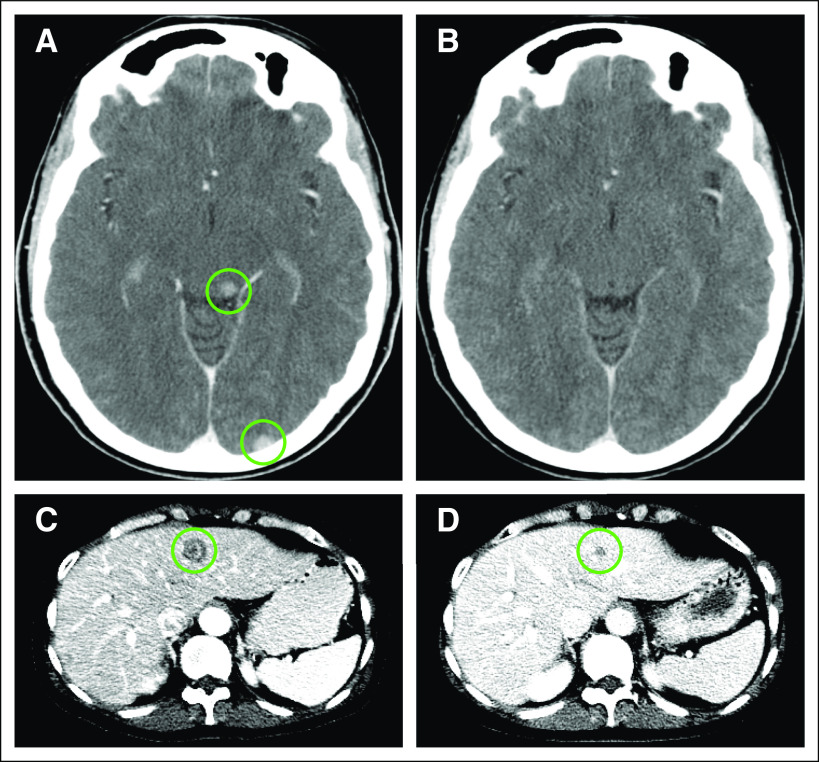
Response to larotrectinib in a patient with a TRK fusion-positive metastatic breast cancer. Baseline axial computed tomography imaging of the brain was performed with the addition of iodinated contrast that showed (A) metastatic brain disease (circles) and after 8 weeks of treatment with larotrectinib, (B) complete response. Radiologic partial response was also evident in the patient’s liver metastases at (C) 8 weeks, which continue at (D) 16 weeks. This partial response was visualized throughout her liver, adrenal, and bone metastases.
